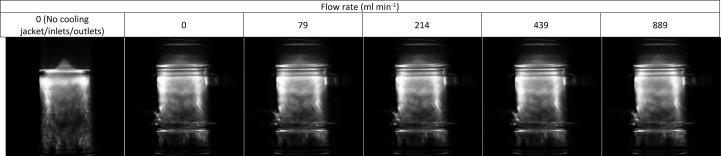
Representative sonoluminescence images for sonolysis of 420 ml of Milli-Q water using 410 kHz ultrasound at 80 W applied power, at liquid flow rates of 0, 0, 79, 214, 439, and 889 ml min^−1^. The image most left shows a reactor without flow, inlets, outlets, or cooling jacket, which enables the standing wave under no flow to be seen more clearly.
